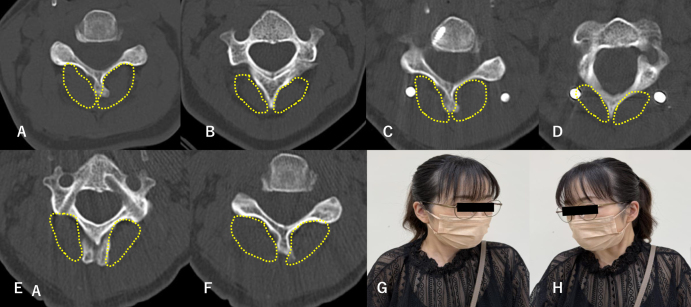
CT images obtained at the C2 and C3 vertebral levels and Clinical photographs of the patient. (A and B) Preoperative axial CT images demonstrating SSC muscle bulk at both C2 and C3 levels. (C and D) Postoperative axial CT images showing that the SSC muscle has been preserved intact at the corresponding levels after fixation surgery. (E and F) Axial postoperative CT images demonstrating intact SSC muscle (yellow line) at C2 and C3 after hardware removal. (G and H) Clinical photographs of the patient performing head rotation, demonstrating recovery of motion without restriction.
